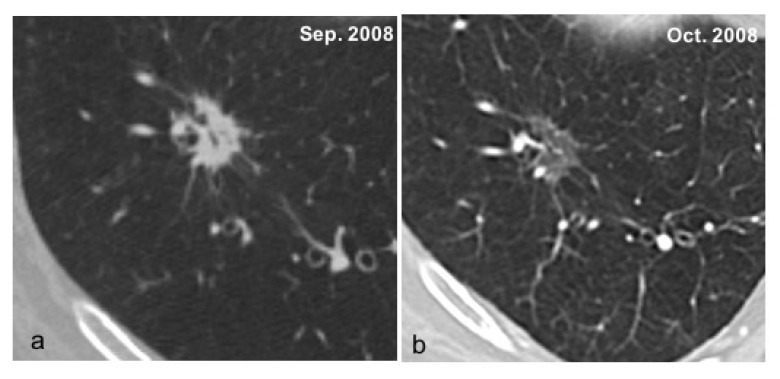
(a,b) This is an example of a solid nodule (a) suspected for malignant lesion. However, at 1-month follow-up CT examination, it tends to disappear due to its inflammatory nature (b).
